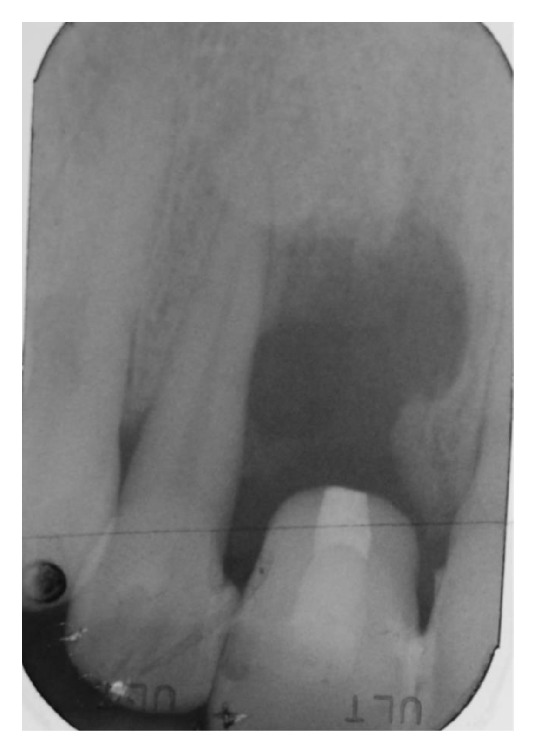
Postoperative X-ray after tooth extraction.
